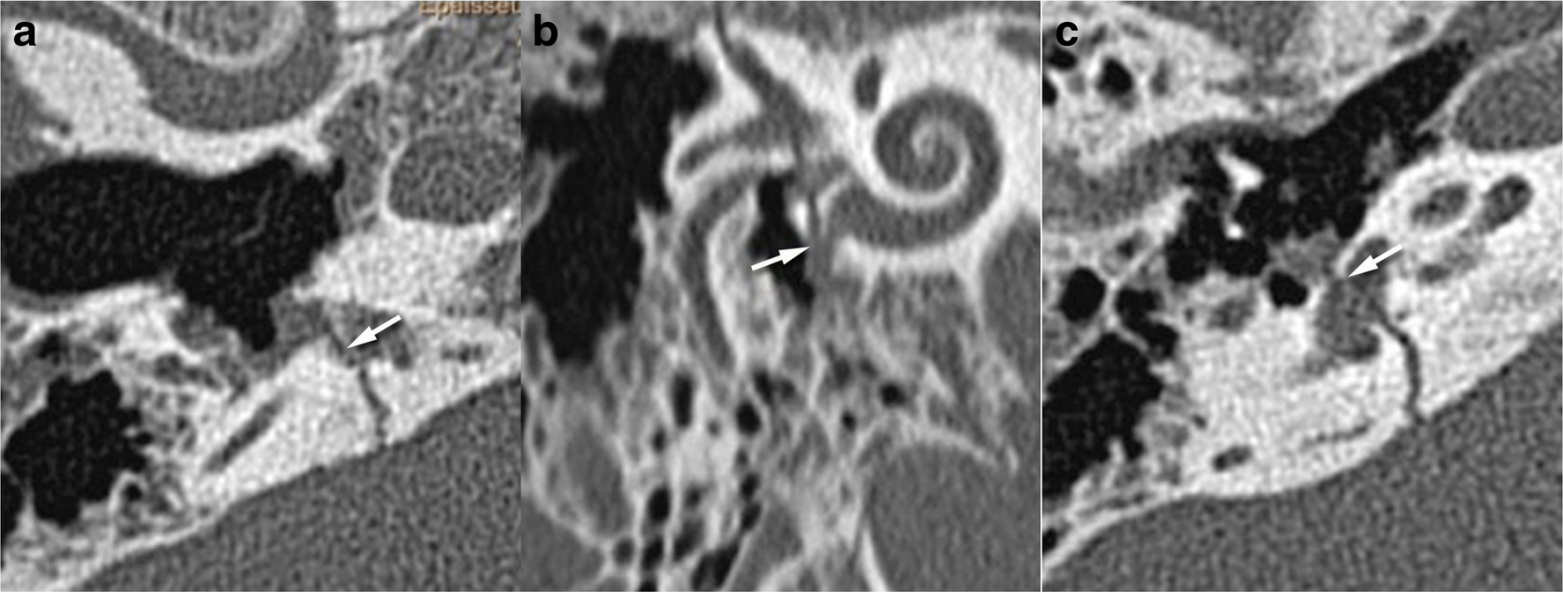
Perilymphatic fistulae: round and oval windows are the two communication routes between the middle ear and inner ear, implying two weakness zones. Axial and oblique CT views showing fractures (*white arrows*) crossing the round (a, b) and oval (c) windows
